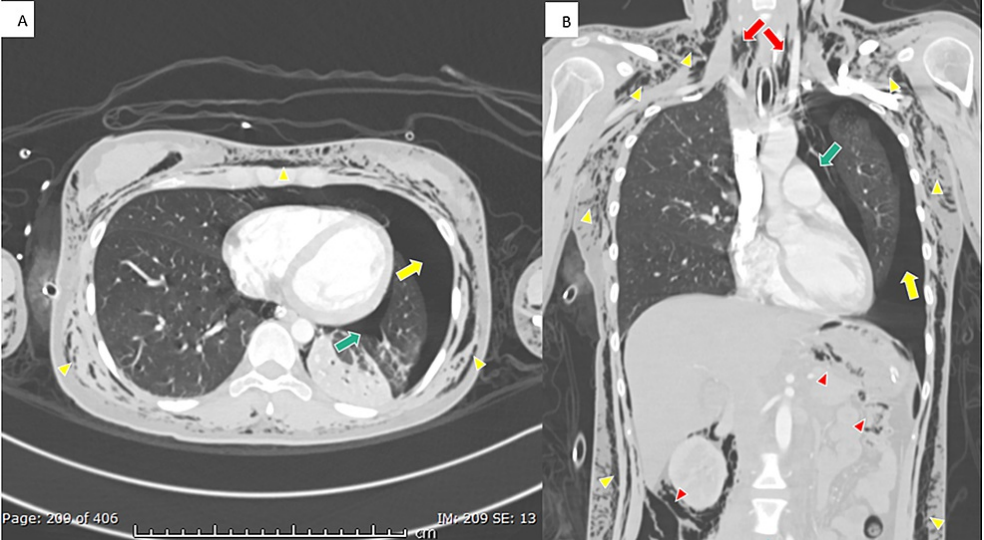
CT chest A) axial and B) coronal CT chest in the lung window showing diffuse subcutaneous surgical emphysema (yellow arrowhead), large left pneumothorax (yellow arrow), moderate amount of pneumopericardium (green arrow), pneumomediastinum (red arrow), and large amount of intra-abdominal air (red arrowhead). CT, computed tomography.
